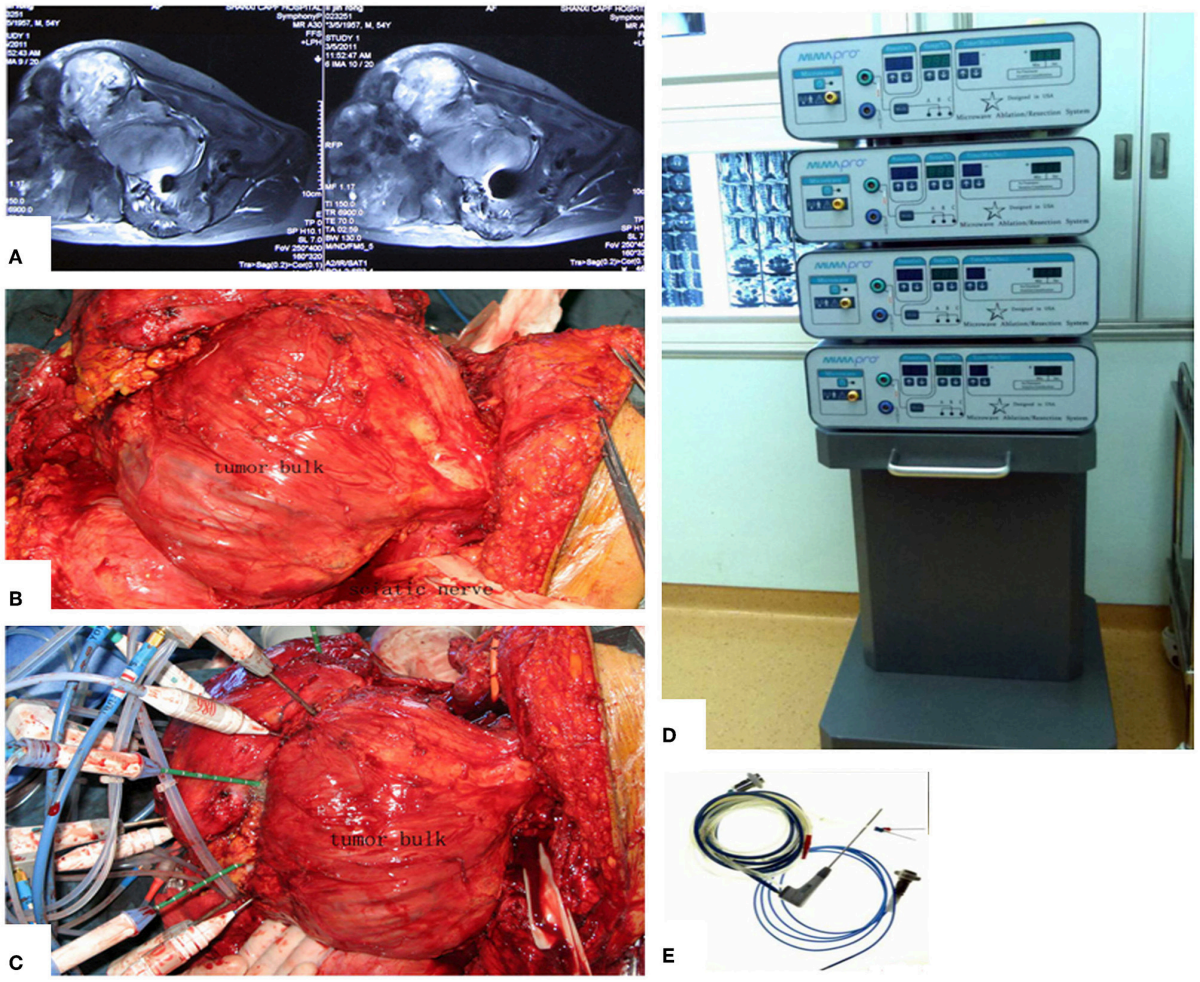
MWA process and microwave generator. (A) MRI shows a massive pelvic malignancy. (B) Exposure and dissection of the tumor mass from surrounding normal tissues. (C) Insertion of the antenna and thermocouples into the tumor mass, usually at the junctional zone of the tumor bulk and ilium bone. (D) Microwave generator. (E) Antenna.
